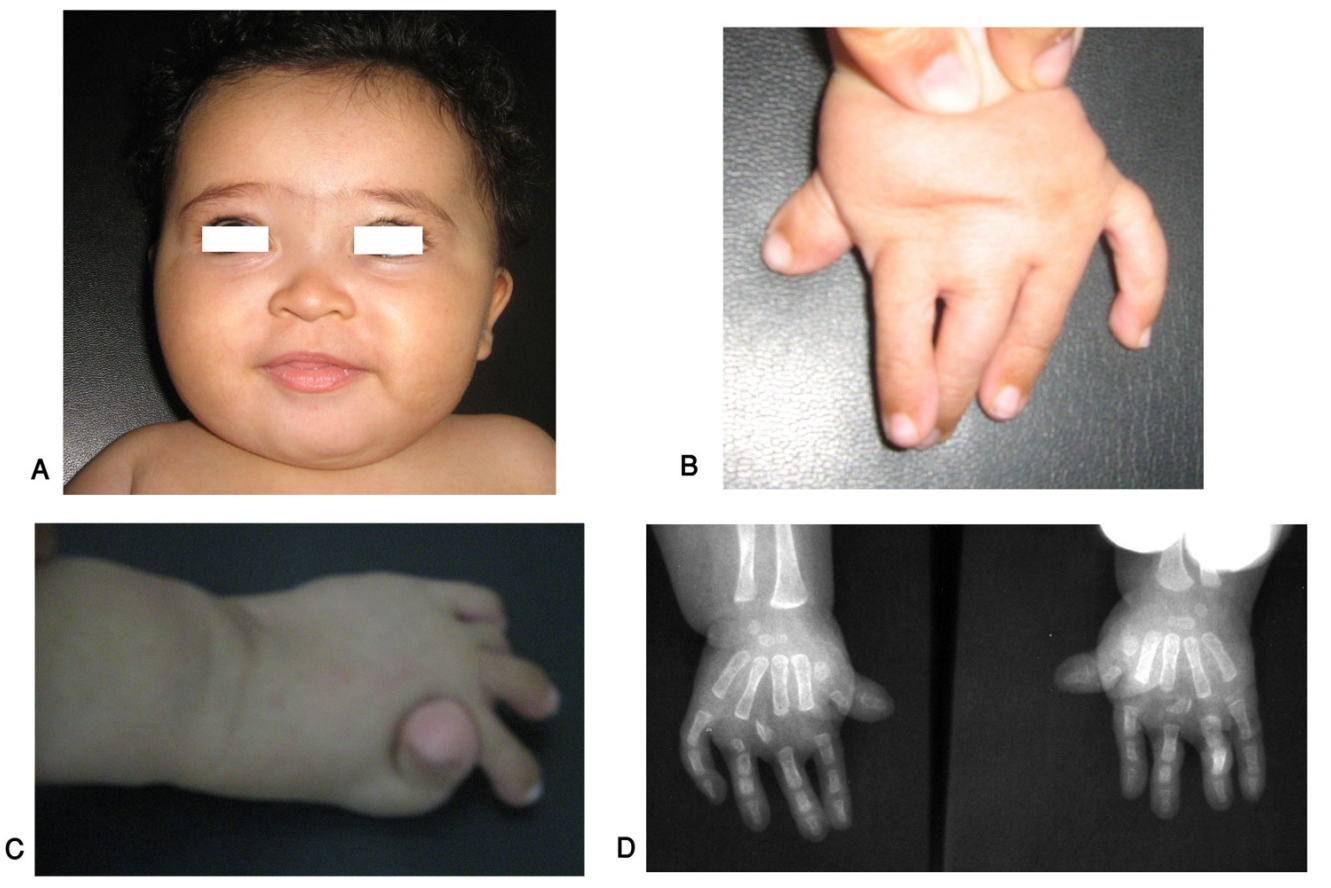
An Egyptian patient with Temtamy preaxial brachydactyly syndrome. Note round flat face (A), preaxial brachydactyly of hand and feet (B, C) and X-ray of both hands showing preaxial brachydactyly and hyperphalangism. (Limb Malformations & Skeletal Dysplasia Clinic, MSU, NRC).
